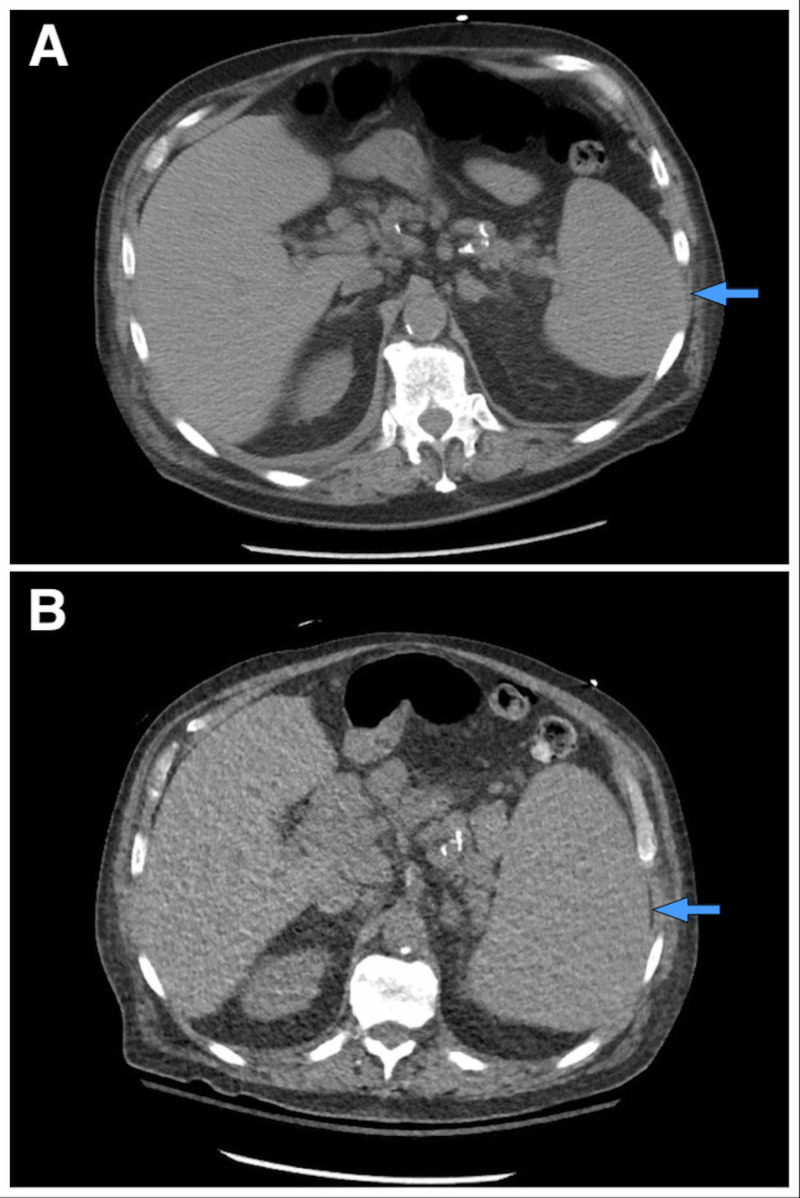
CT scan of the abdomen The image shows a normal-sized spleen (blue arrows) 10 months prior (A) in comparison to newly-found prominent splenomegaly (B) during his current hospitalization CT: computed tomography
